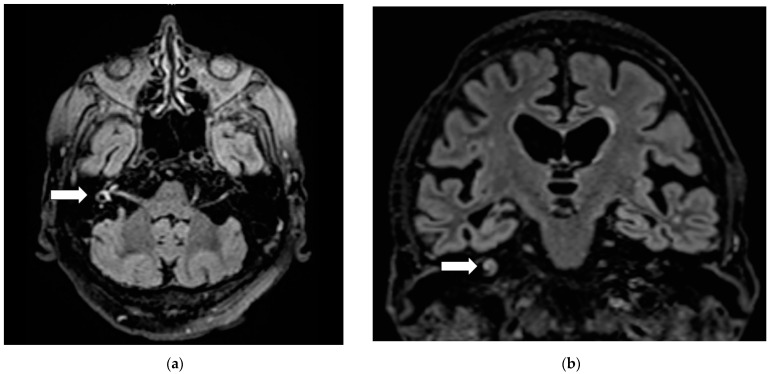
Right labyrinthitis: Hyperintensity (arrows) on axial (a) and frontal (b) FLAIR sections in right vestibule, semicircular canals and cochlear.
